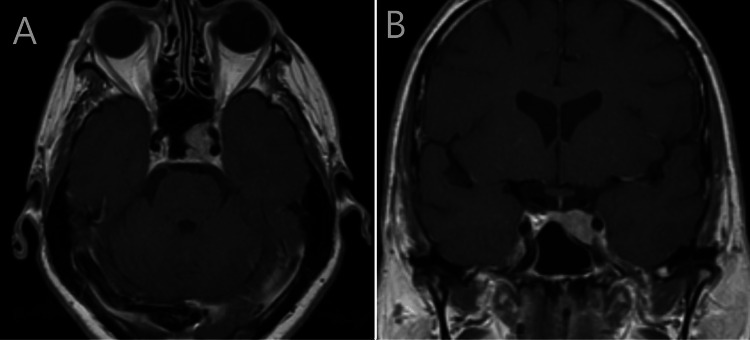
Initial imaging five years prior to visual symptoms Axial (A) and coronal (B) T1-weighted gadolinium-enhanced MRI showing the left parasellar lesion when it was initially discovered, five years before the patient became symptomatic. Imaging shows a homogenous enhancing lesion centered on the left cavernous sinus MRI: magnetic resonance imaging
